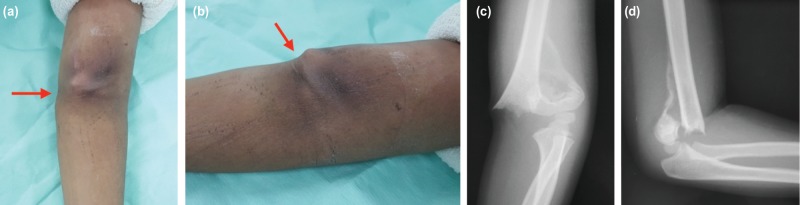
(a, b) shows the metaphyseal spike (red arrow) obtruding the skin at the medial aspect of the antecubital fossa. Brachial and radial pulses are not palpable clinically, and there is no signal detected on hand-held Doppler examination. (c, d) Plain radiographs of the affected left elbow reveal a Gartland III supracondylar humeral fracture with callus formation at the posterior aspect of the proximal fracture fragment.
